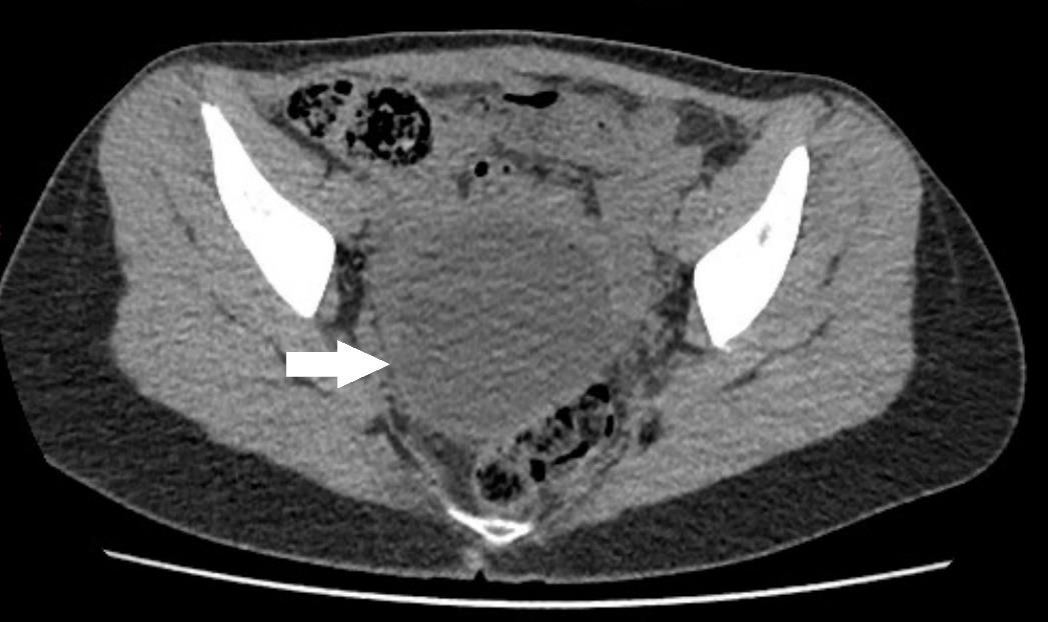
Non-contrast CT scan of pelvis showing cystic image in the retro-uterine region on the right (white arrow).
